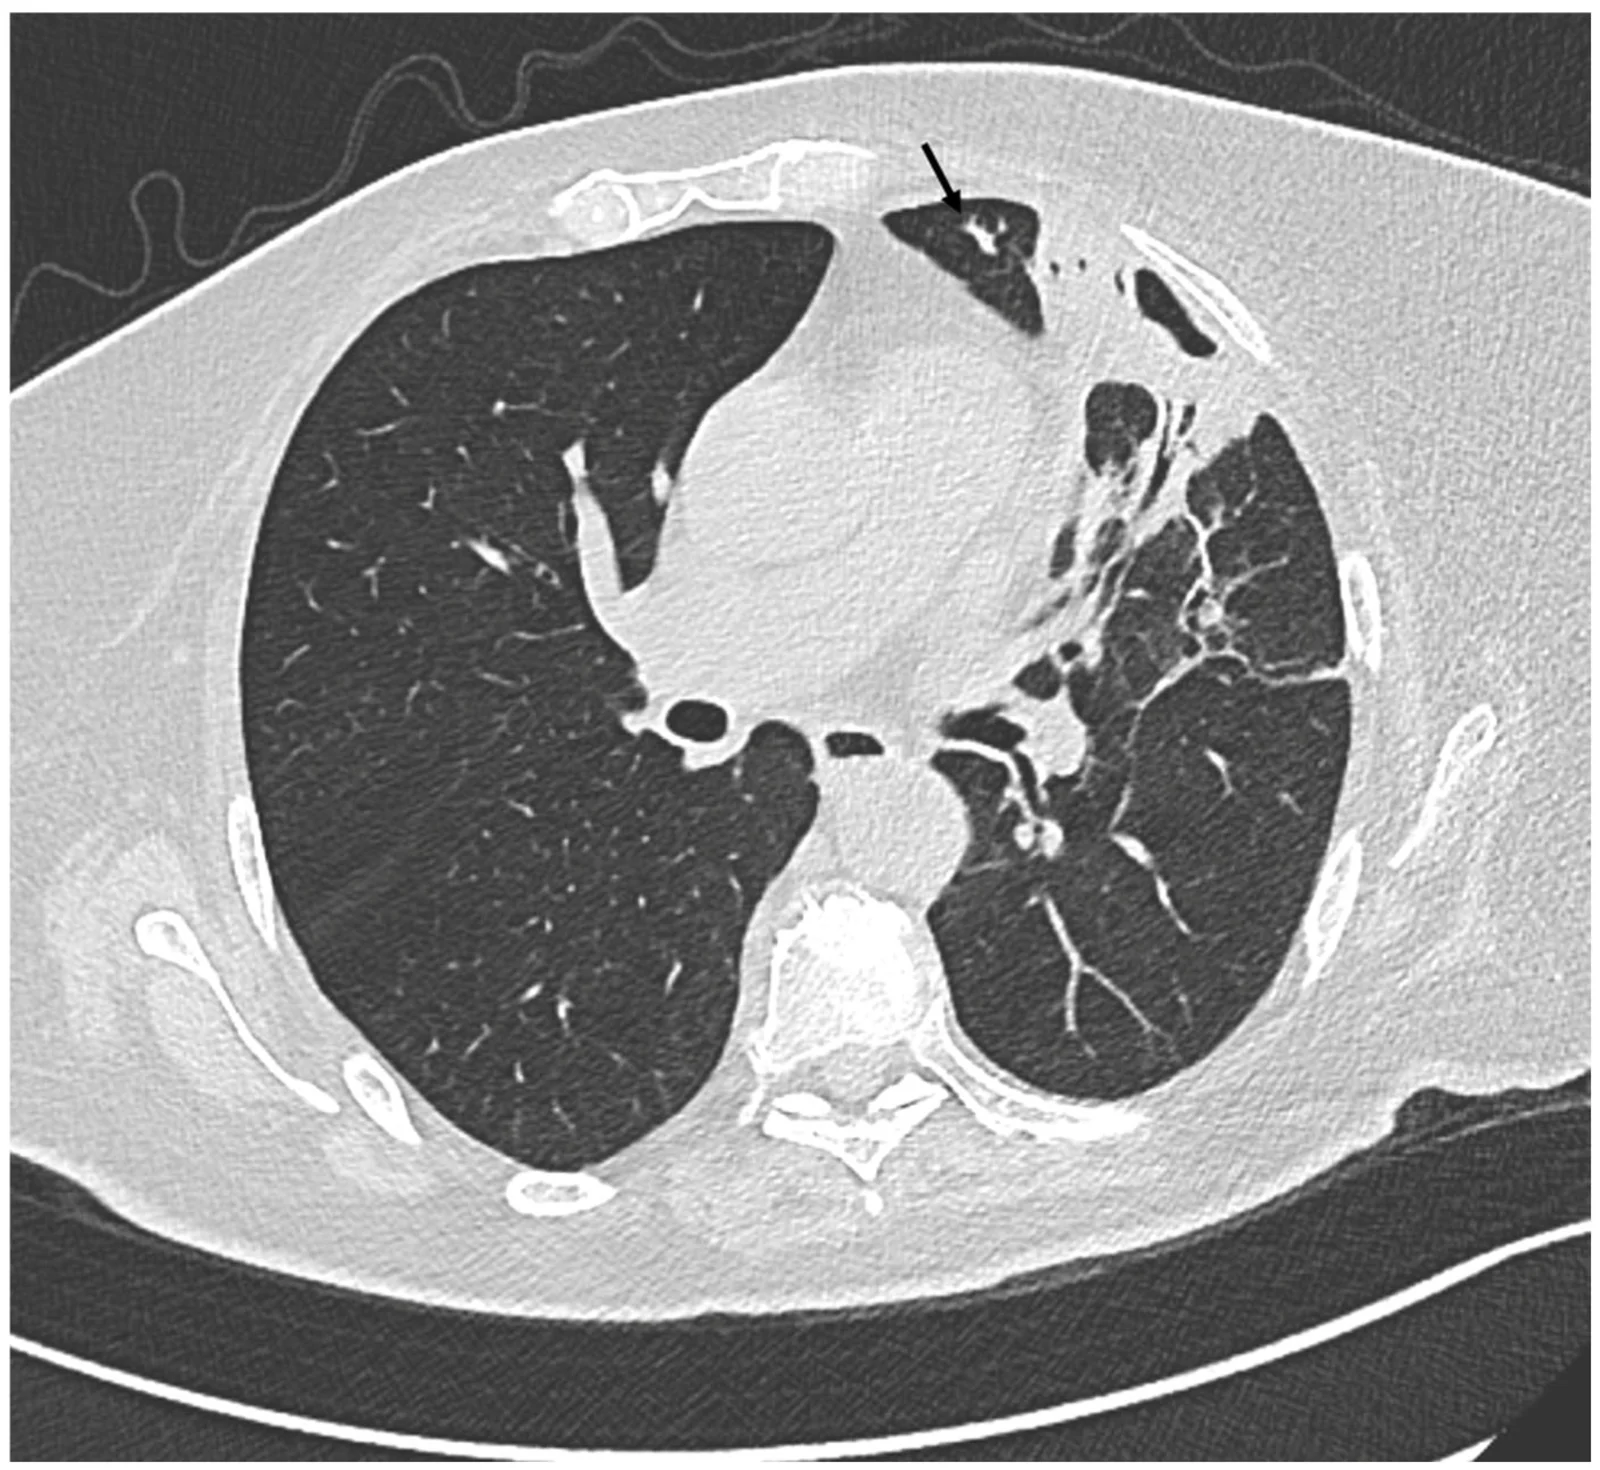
HRCT, axial plane. Case of an acute Q fever pneumonia: HRCT shows an area of airspace consolidation in the inferior lingular segment, accompanied by interstitial thickening and nonspecific parenchymal nodules (black arrow).
